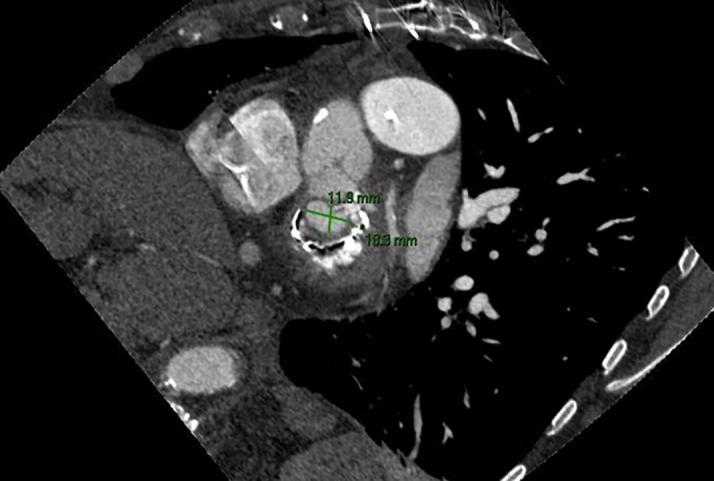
Gated Cardiac Computed Tomography With Mitral Annular Dimensions 2 × 1.8 cm
